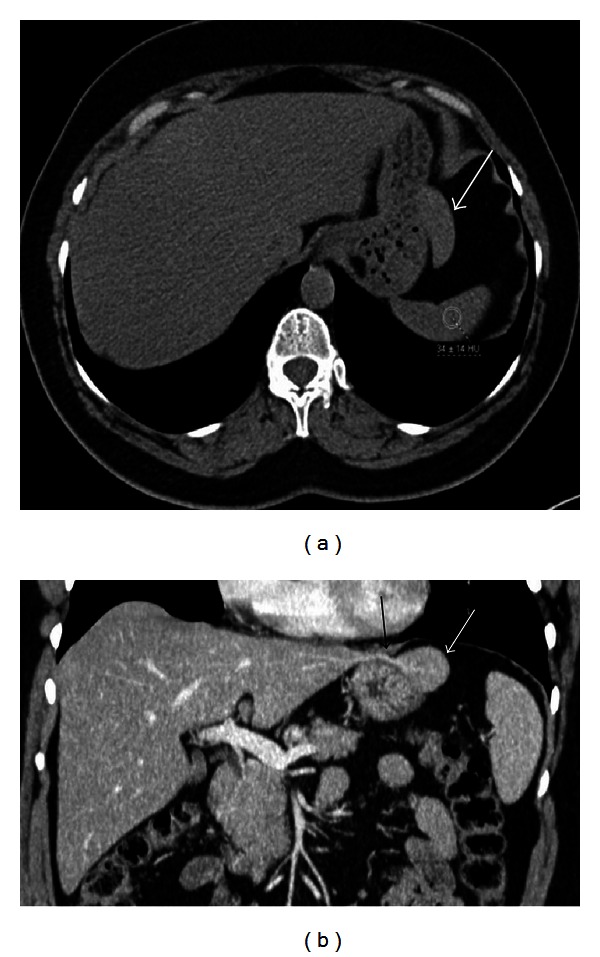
Nonenhanced axial CT image (a) of a 48-year-old female patient reveals a soft tissue mass (arrow) nearby greater curvature of the stomach. On coronal reformatted contrast enhanced CT image, there is a stalk (black arrow) between left lobe of the liver and the mass (white arrow) indicating accessory liver.
